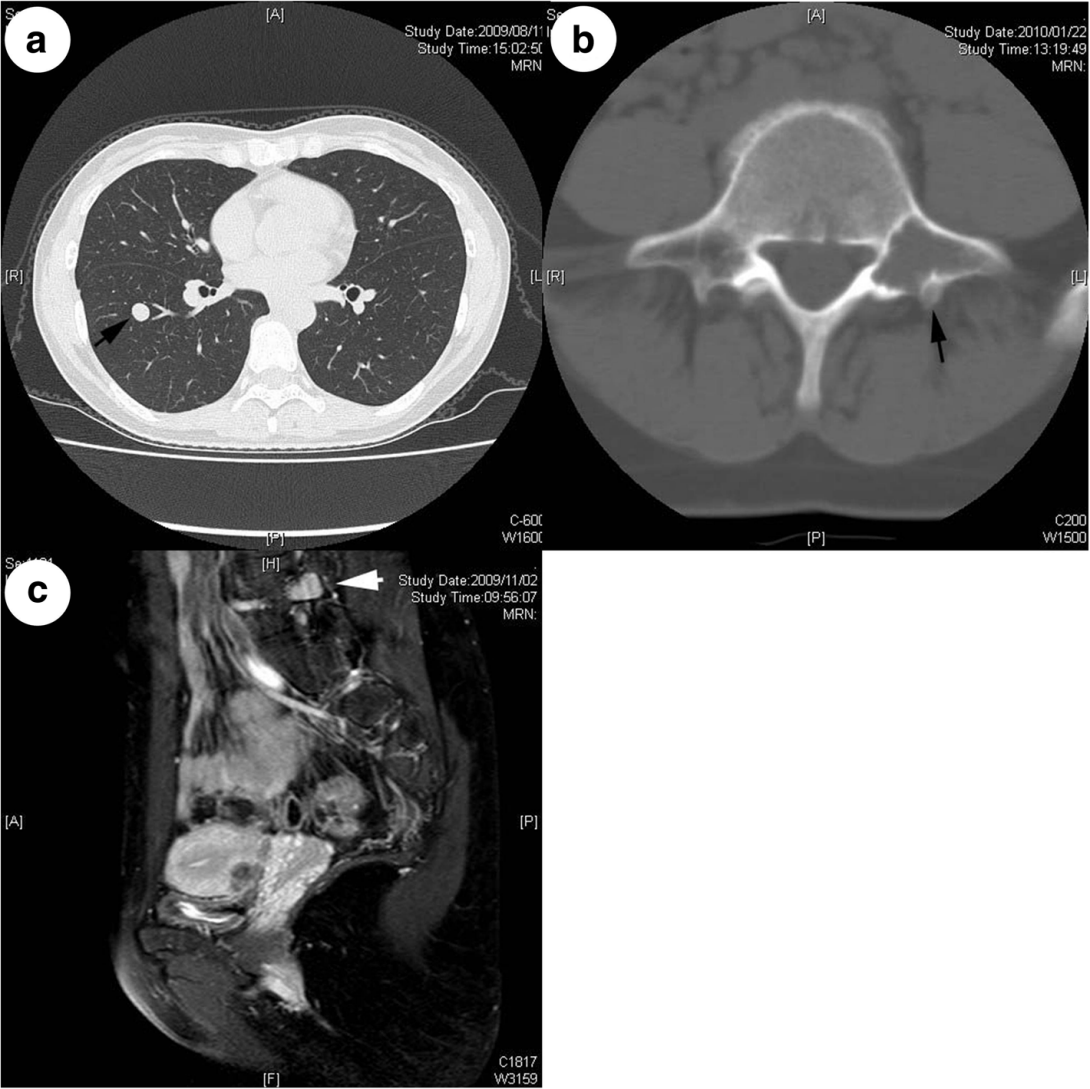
a Computed tomographic scan showing a well-circumscribed nodule in the right lung (arrow). b Magnetic resonance (MR) image showing a lytic lesion in the left transverse process in the fifth lumbar vertebra (arrow). The lesion appeared to erupt to the posterior surface of the transverse process. No mass lesion was found in the spinal canal. c Sagittal MR image of the lumbar region. A mass lesion was situated posterior to the lumbar vertebral column (arrow)
